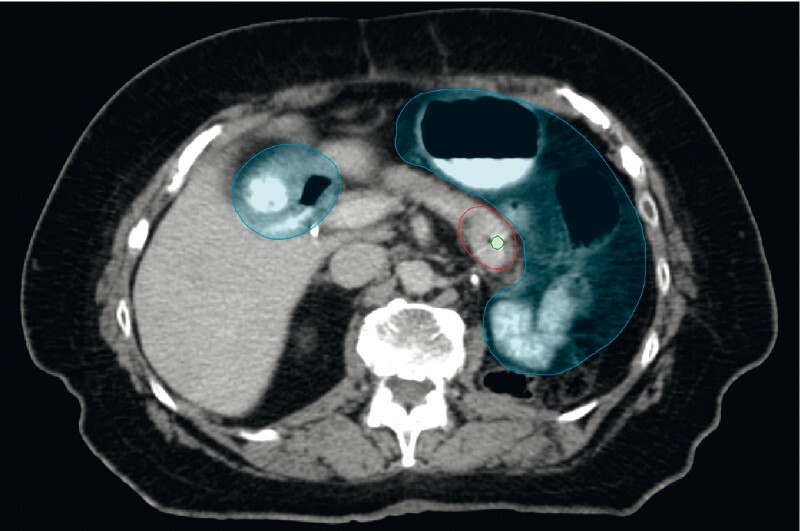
Computed tomography image used for radiotherapy planning showing the fiducial marker (encircled in green) implanted in the tumor of the pancreatic tail (encircled in red). Organs at risk (stomach, bowel) are marked in blue.
